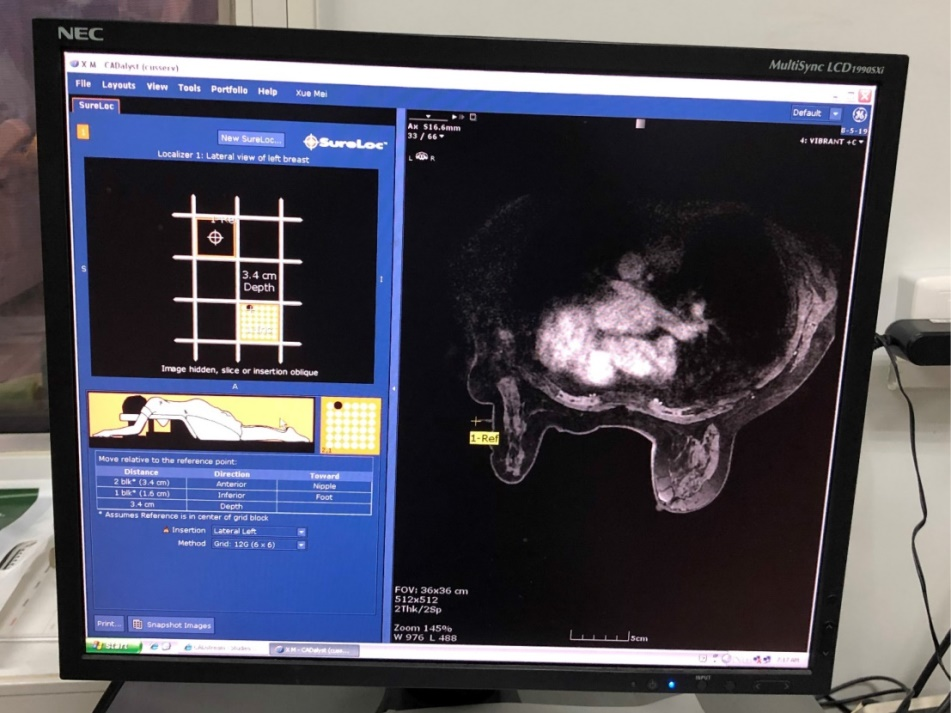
Computer-aided targeting in a 47-year-old woman with right breast lesion which is identified in the computer-aided detection (CAD) system. The CAD system provides a graphic depicting the optimal entry point for the needle (yellow crosshairs), and it also calculates coordinates of the lesion towards the nipple and foot. Especially, the lesion depth is important for MRI-guided intervention.
